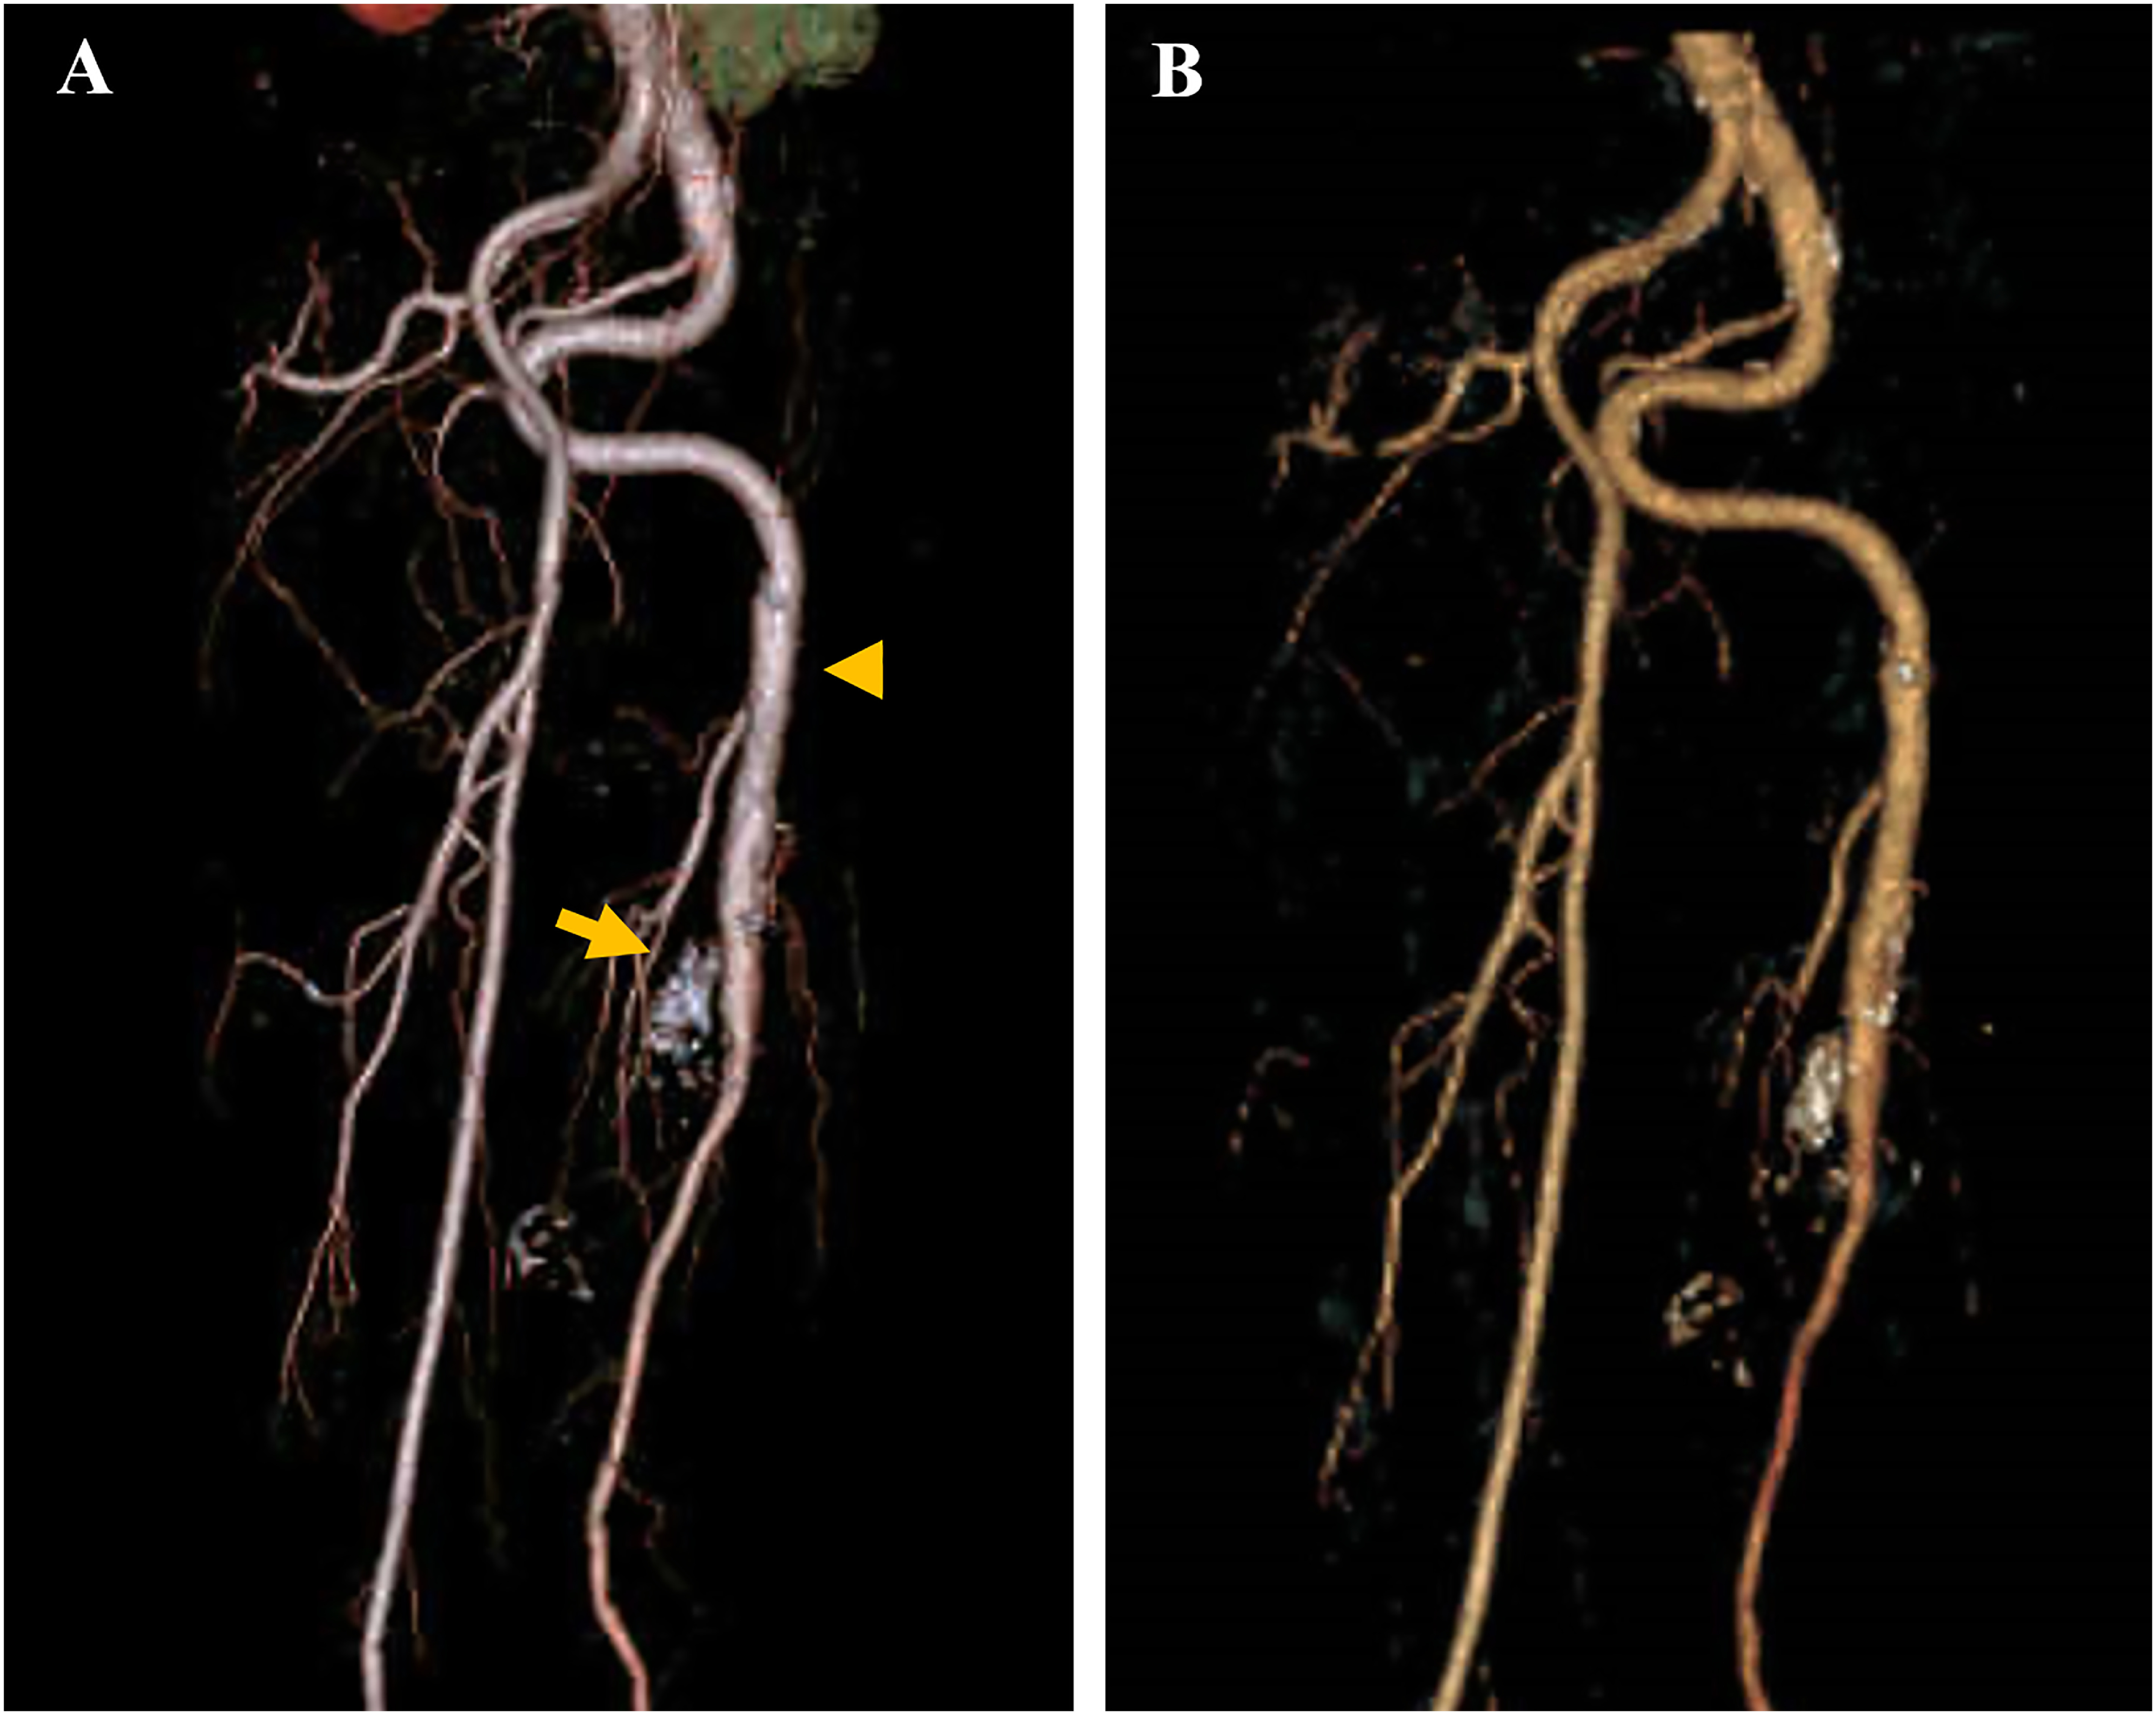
Fig. 3 Postoperative computed tomography angiography. (A) Postoperative image shows the absence of arteriovenous fistula (arrow), but notably persistent dilation of the left femoral artery (arrowhead). (B) Two years thereafter, the femoral artery did not change postoperatively.
